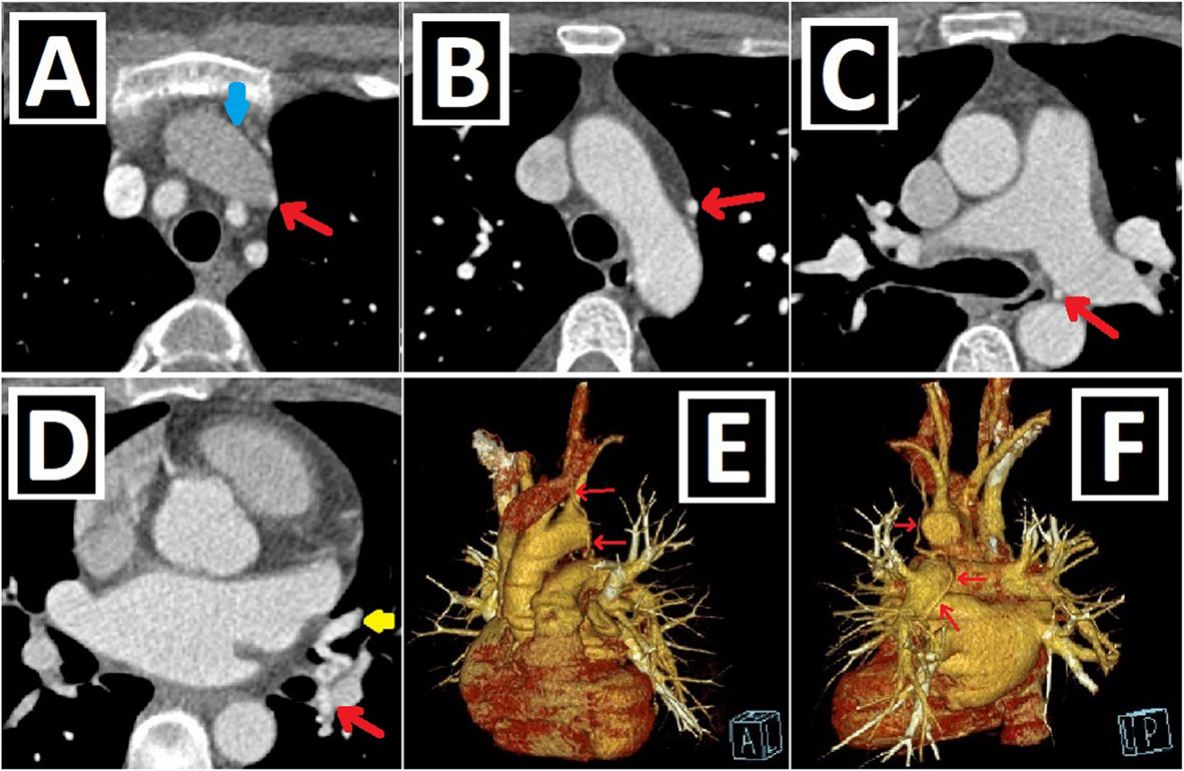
Levoatriocardinal vein-1. a–f CT images of a patient with mitral valve stenosis due to acute rheumatic fever. The first four axial CT images (a–d) depict the venous structure (red arrows) coursing between the left brachiocephalic vein (blue arrow) and the left upper pulmonary vein (yellow arrow). The density difference between the cranial and caudal ends of the vessel suggests that flow direction is caudo-cranial. 3D VRT CT images with anterior and posterior views (e, f) show the course of the vessel. This vascular structure observed in the posterior of the pulmonary artery in the patient with mitral stenosis, which is a LOL, is compatible with LACV (red arrows)
